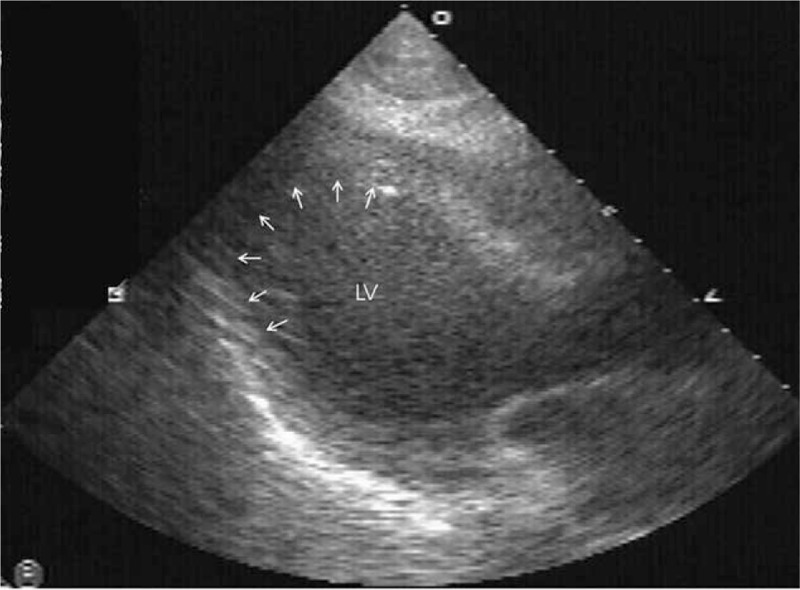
Portable transthoracic echocardiograph (parasternal long axis view) showing typical ballooning of the apex in the heart during systole. LV = left ventricle.
